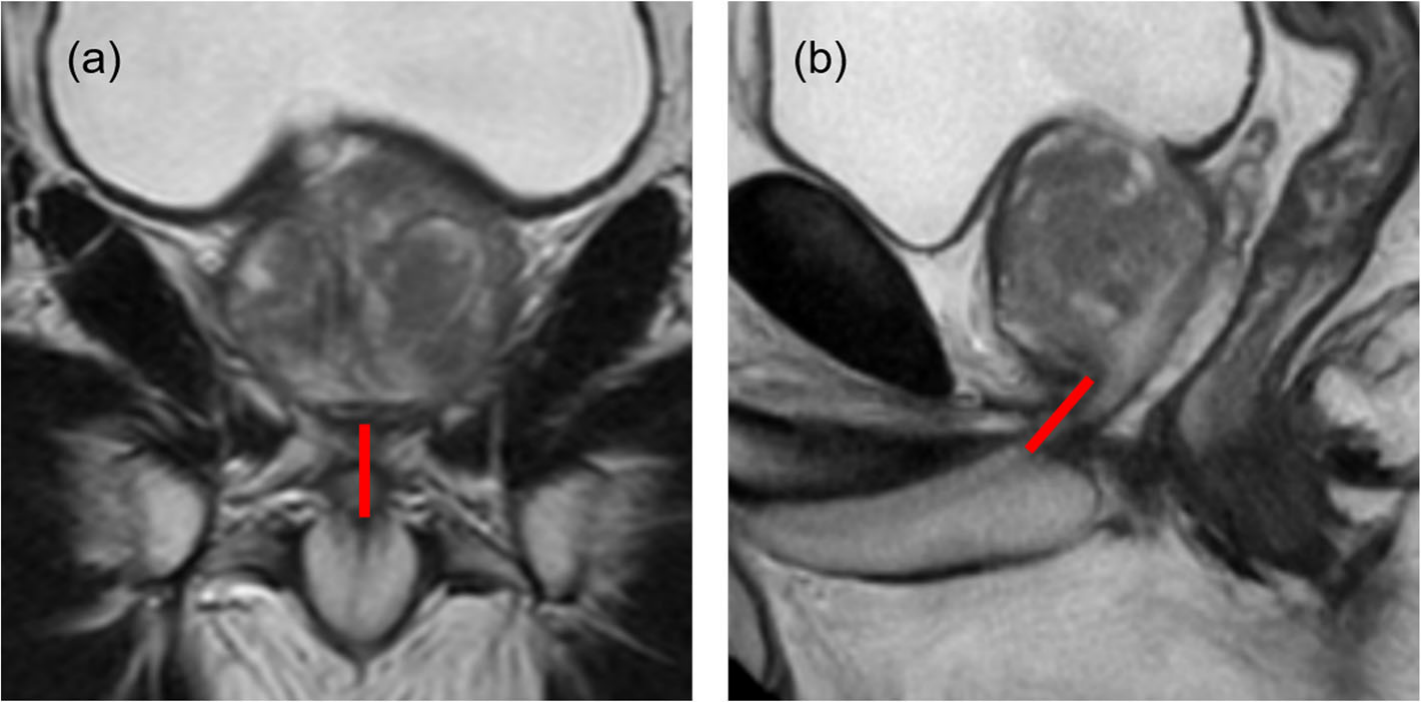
The preoperative membranous urethral length as the distance from the prostatic apex to the level of the urethra at the penile bulb is measured in the T2-weighted MRI a coronal and b sagittal planes
